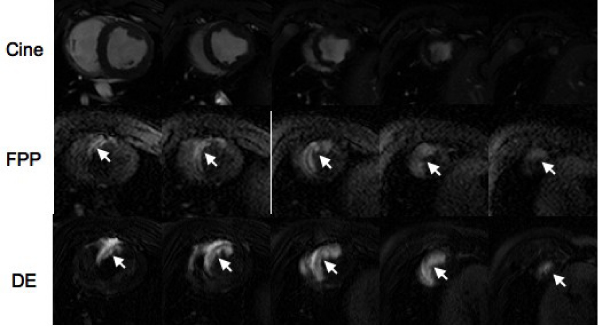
Multislice MR images showing the LV on cine (top) and the LAD perfusion territory on corresponding FPP (middle) and early CE (bottom) MRI as hyperenhanced regions.
